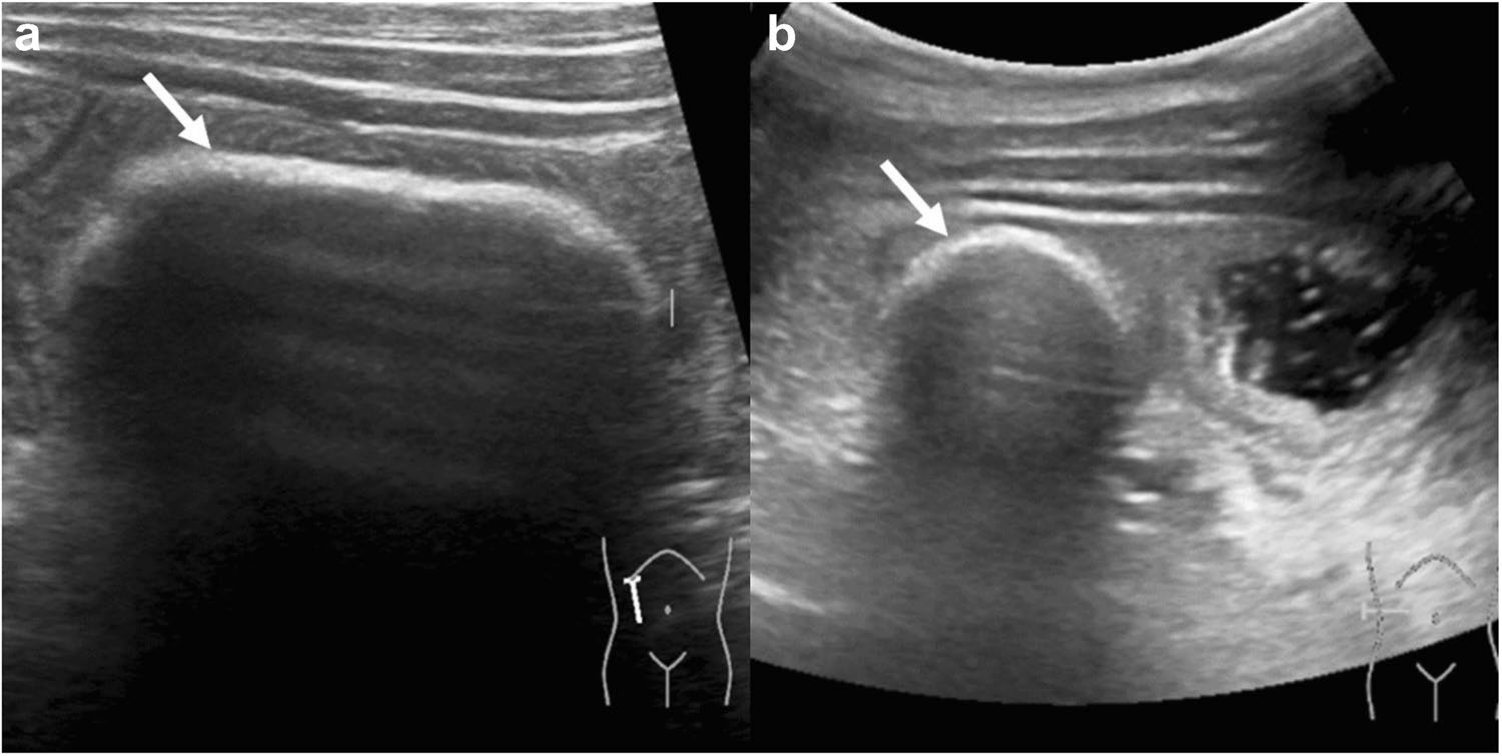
Abdominal ultrasound of a 5-year-old girl with a percutaneous transhepatic biliary drainage with associated stone formation. Abdominal ultrasound was performed to correlate the magnetic resonance imaging finding (see Fig. 1). a Longitudinal and (b) transverse ultrasound shows the stone at the biliary drainage tip with hyperechoic stone outline (*arrows*) and posterior acoustic shadowing
